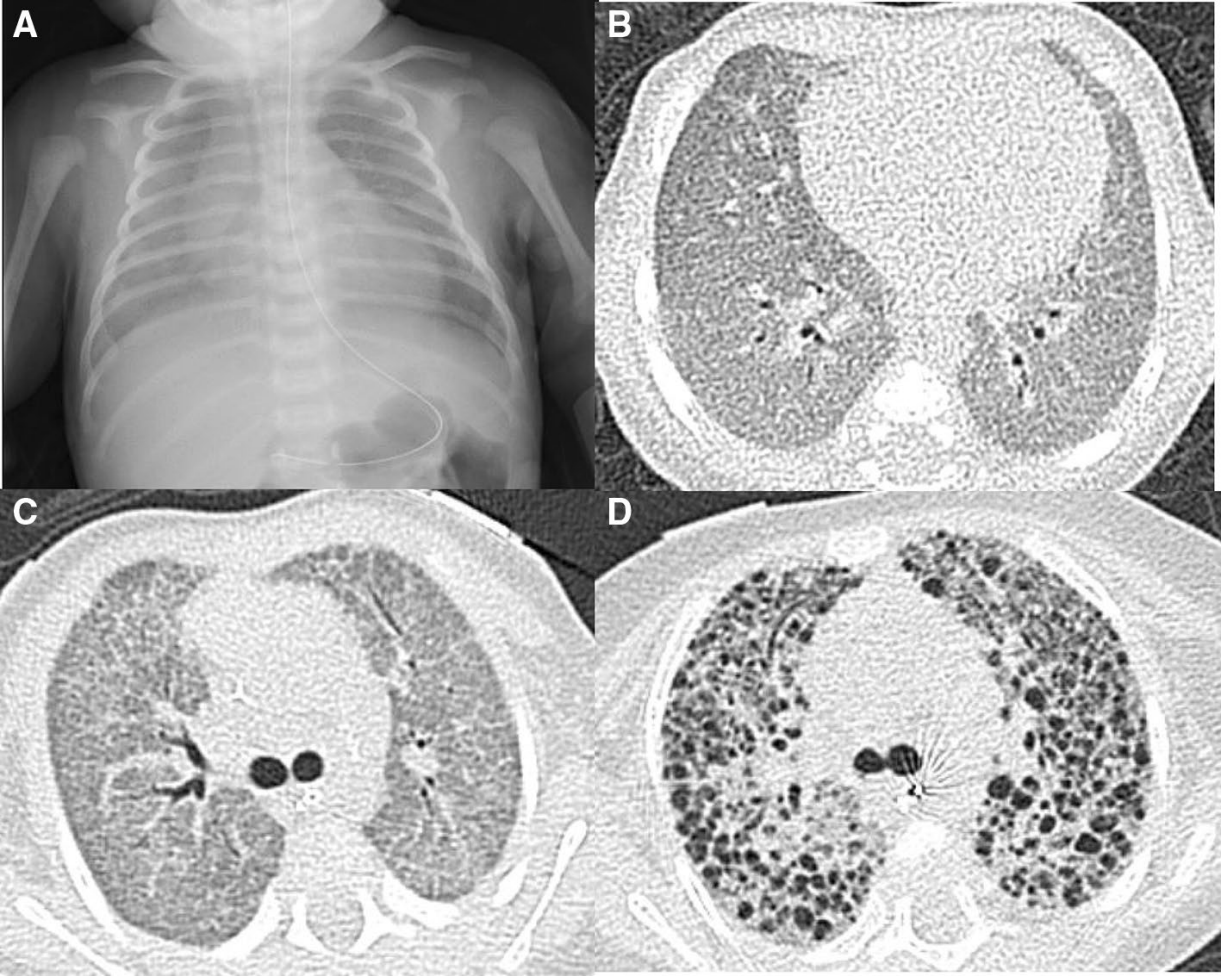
A severe manifestation of SP-C deficiency. A 1-month-old infant with failure to thrive and hypoxemia (70% of SpO_2_). A,B CXR and CT at age 1 month showed diffuse haziness and GGOs, respectively. The imaging findings look like those of respiratory distress syndrome in premature newborns. C CT at age 2 months showed thickening of interlobular septa and mild bronchial dilatation in addition to GGOs. D CT at age 4 months revealed development of multiple emphysematous cysts and progressive overinflation of the lung. The infant succumbed shortly after the CT examination
